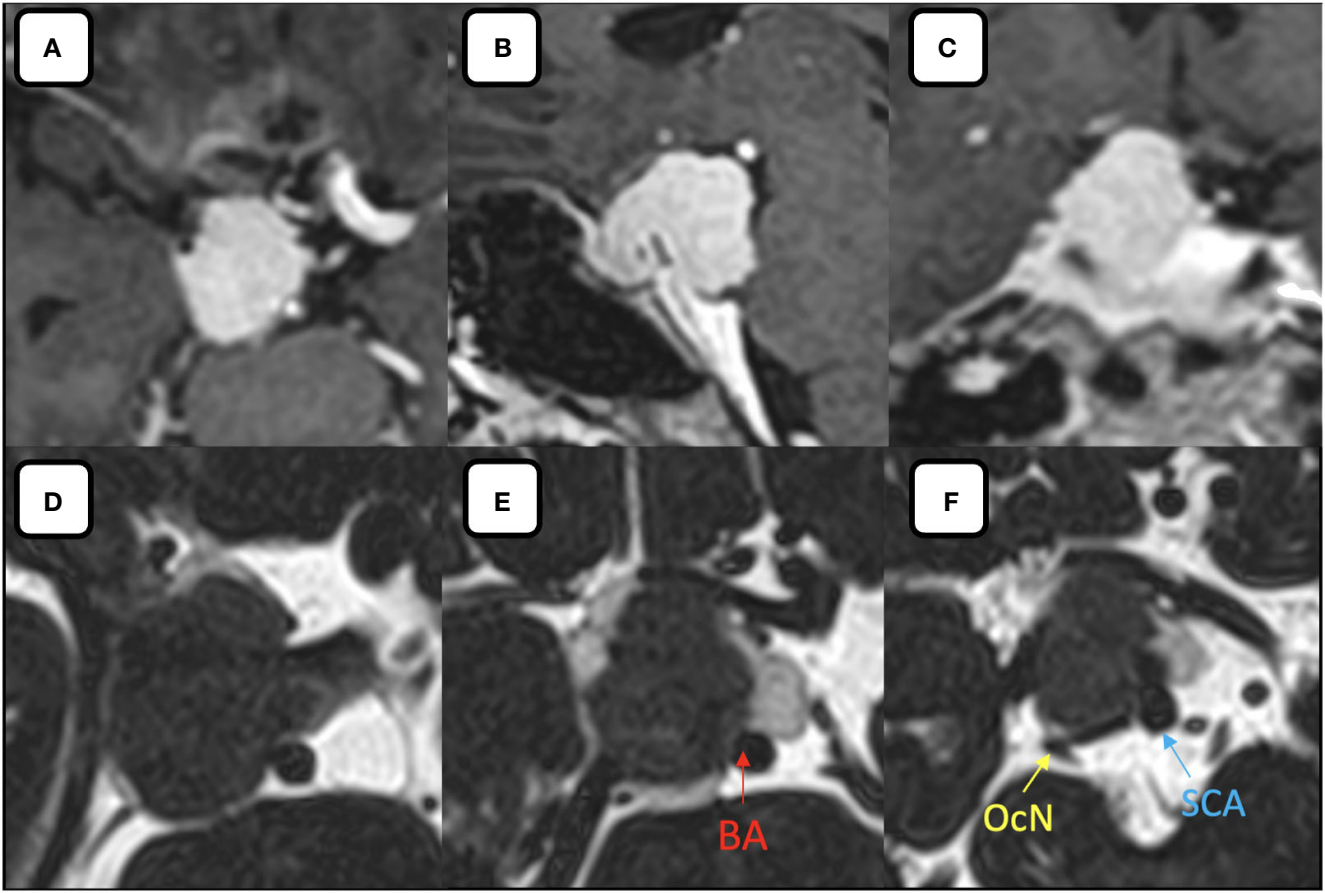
(A–C) Post contrast (A) axial, (B) sagittal, (C) coronal MR images showing the homogenous enhancement of the lesion relative to its surrounding structures. (D–F) Axial MR-Cisternography denotes the anterior displacement of the ICA, partial encasement of the basilar artery by the tumor, postero-lateral displaced oculomotor nerve and superior cerebellar artery by the tumor. BA, basilar artery; OcN, oculomotor nerve; SCA, superior cerebellar artery.
